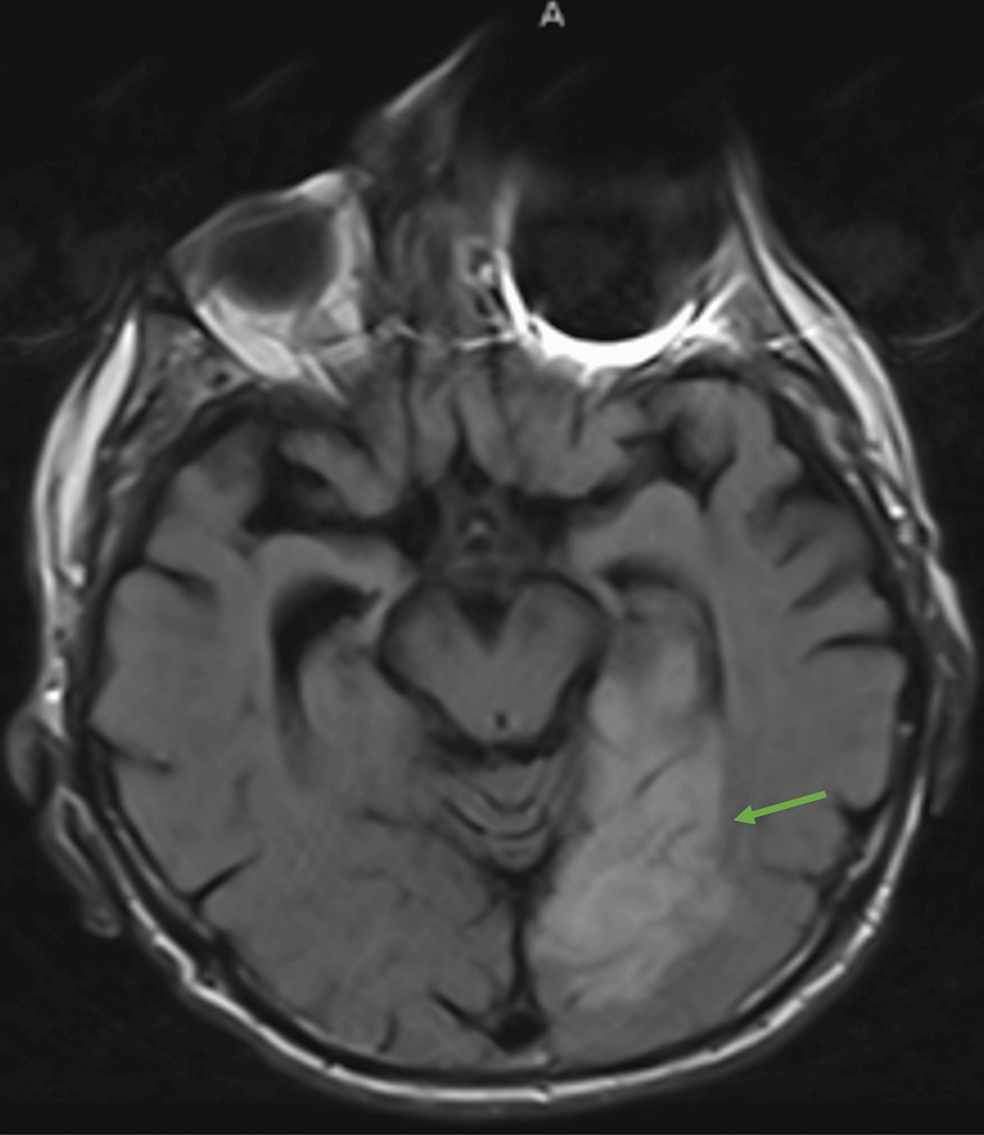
Brain MRI fluid-attenuated inversion recovery (FLAIR) scan (transverse view) showing an acute stroke within the territory of the left posterior cerebral artery (green arrow)
